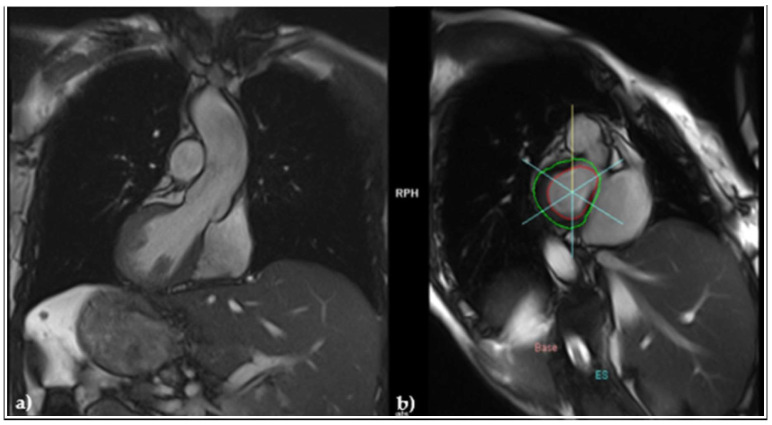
MRI (a) frontal view revealing normally sized cardiac cavities; (b) sagittal view evidencing and the absence of adipose deposits.
